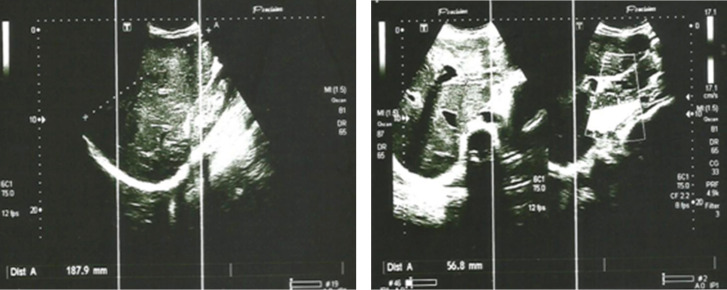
Échographie abdominale : hépatomégalie à contours micro-bosselés avec hypertrophie du segment I (A et B) en rapport avec des stigmates d'hépatopathie chronique débutante Abdominal ultrasound: hepatomegaly with micro bumpy contours with hypertrophy of segment I (A and B) related to signs of early chronic liver disease
